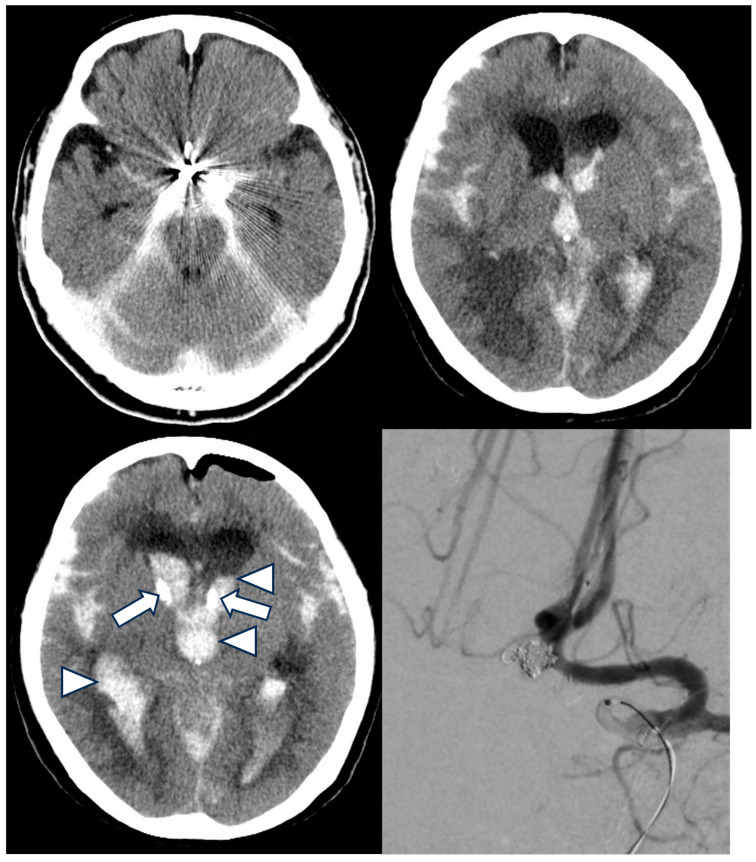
Postprocedural hemorrhagic progression and additional treatment. From upper left to lower right, immediate computed tomography (CT) shows basal cisternal subarachnoid hemorrhage (SAH) and intraventricular hemorrhage (IVH), follow-up CT after anisocoria shows progression of IVH with acute hydrocephalus, CT after bilateral external ventricular drainage (EVD) shows persistent IVH after emergency cerebrospinal fluid diversion, and next-day angiography shows additional trans-cell coiling. Because persistent aneurysmal bleeding could not be excluded on the basis of CT progression, additional trans-cell coiling was performed on the following day. White arrowheads indicate representative IVH, and white arrows indicate the bilateral EVD catheter trajectories/tips on the post-drainage CT image.
